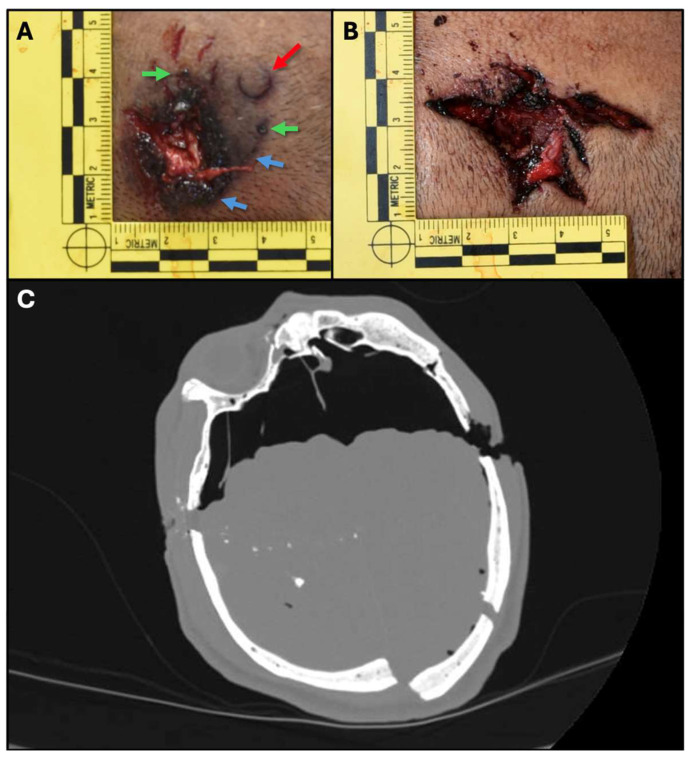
Cranial Gunshot Wounds and CT Scan of Case 2. (A) Entrance wound caused by Bullet A, located on the right temporal region of the head. The wound is irregularly oval with stellate margins, featuring three small notches, and measures approximately 1.6 cm by 1.2 cm. The wound exposes brain tissue, with a visible protruding flap of dura mater and a surrounding dark brownish discoloration measuring 3.2 cm by 3.5 cm. Key forensic indicators are present, highlighted by colored arrows: Werkgartner’s sign is indicated by a red arrow, showing the imprint of the pistol’s recoil spring guide rod; a burn halo and soot deposition are marked by blue arrows; and unburned powder residues are identified with green arrows around the wound; (B) Exit wound caused by Bullet B on the left occipital region. This wound is characterized by hemorrhagic, thickened, and irregular stellate margins with six distinct arms, measuring approximately 5.3 cm by 3.5 cm, exposing underlying skull and brain tissue; (C) CT scan showing intracranial bone fragments in a “comet tail” pattern, characteristic of an entrance wound. The scan provided crucial information to differentiate between entrance and exit wounds, supporting the conclusion that Wound A was the entrance wound while Wound B was the exit wound.
